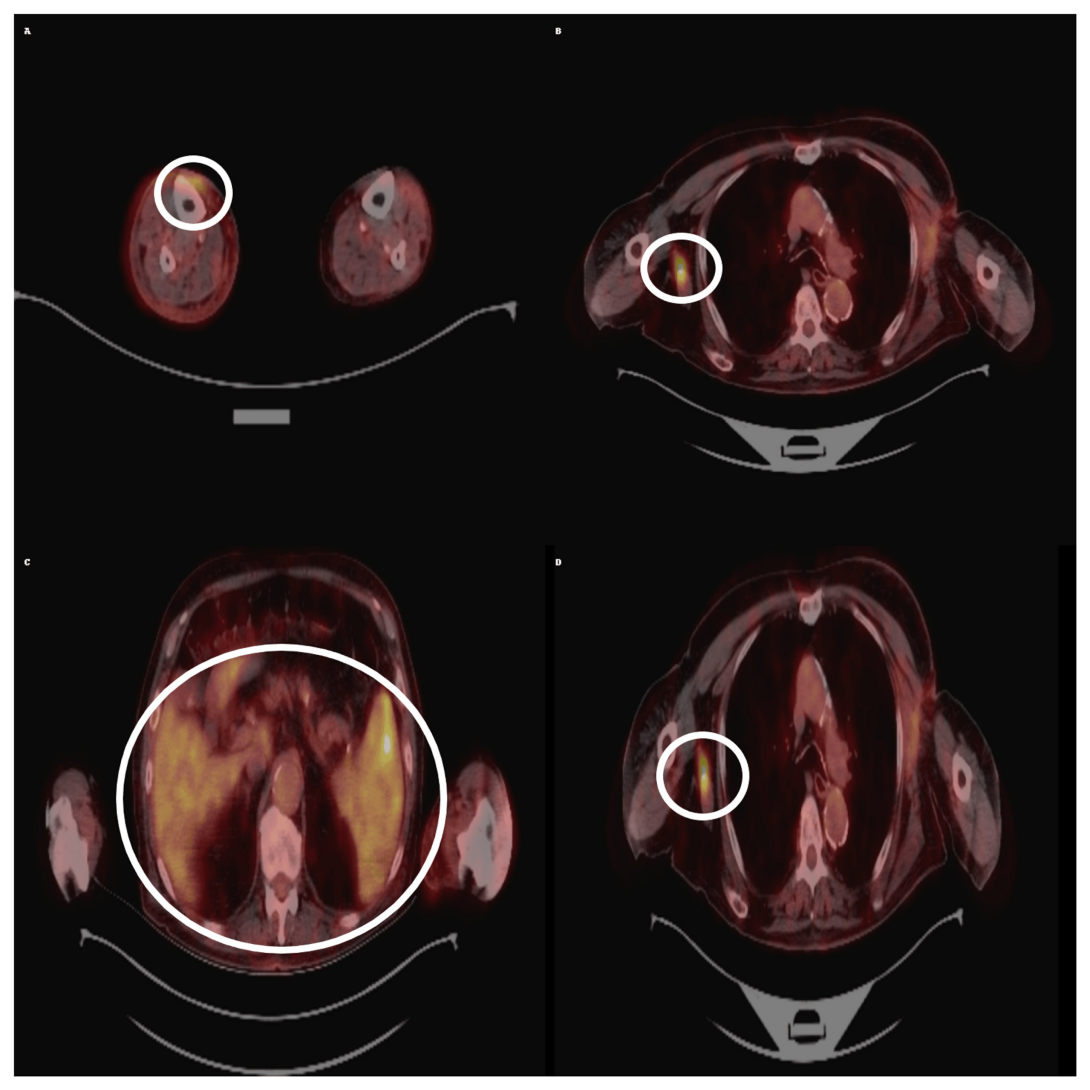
PET/CT scan in November 2024 showing disease progression with new hypermetabolic lesions. Axial fused PET/CT images (A-D) demonstrate new hypermetabolic lesions in the extremities, mediastinum, and spleen. The regions of interest are indicated by white ellipses, with SUVmax increasing up to 15.5, consistent with aggressive Kaposi sarcoma activity.
